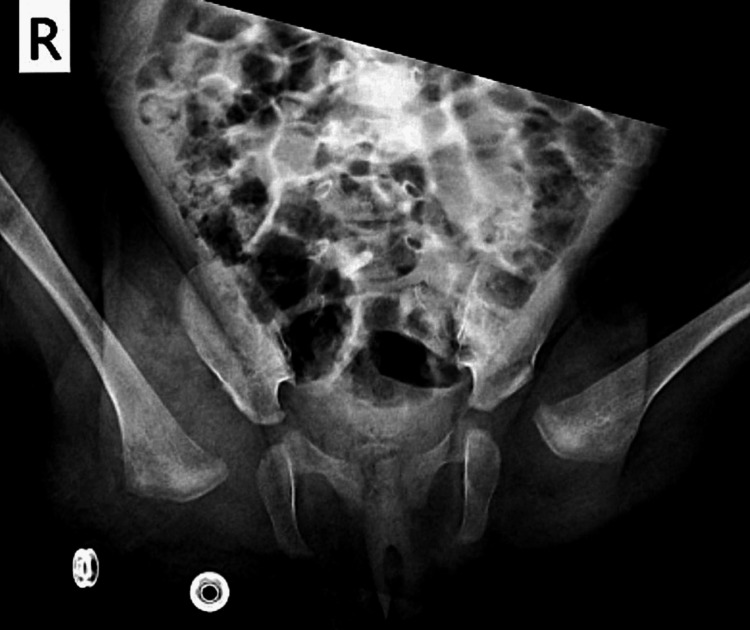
Anteroposterior pelvic radiograph demonstrating obturator (inferior) dislocation of the right hip
